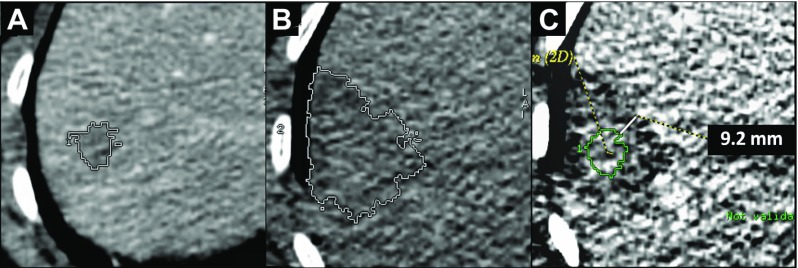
42-year-old man with a liver metastasis of a melanoma in segment VII and ablation with complete tumor coverage. a The focal hypovascular metastasis with the corresponding segmentation on the pre-ablation ce-CT image. b The ablation zone after 24 h with the corresponding segmentation. c The segmentation of the metastasis projected on the post-ablation ce-CT image with the measurement of the smallest distance (9.2 mm) to the boundary of the ablation zone (Category A). No LTP during a follow-up period of 5 years. The term ‘not validated’ in the figures corresponds to the fact that the software is not commercially validated
